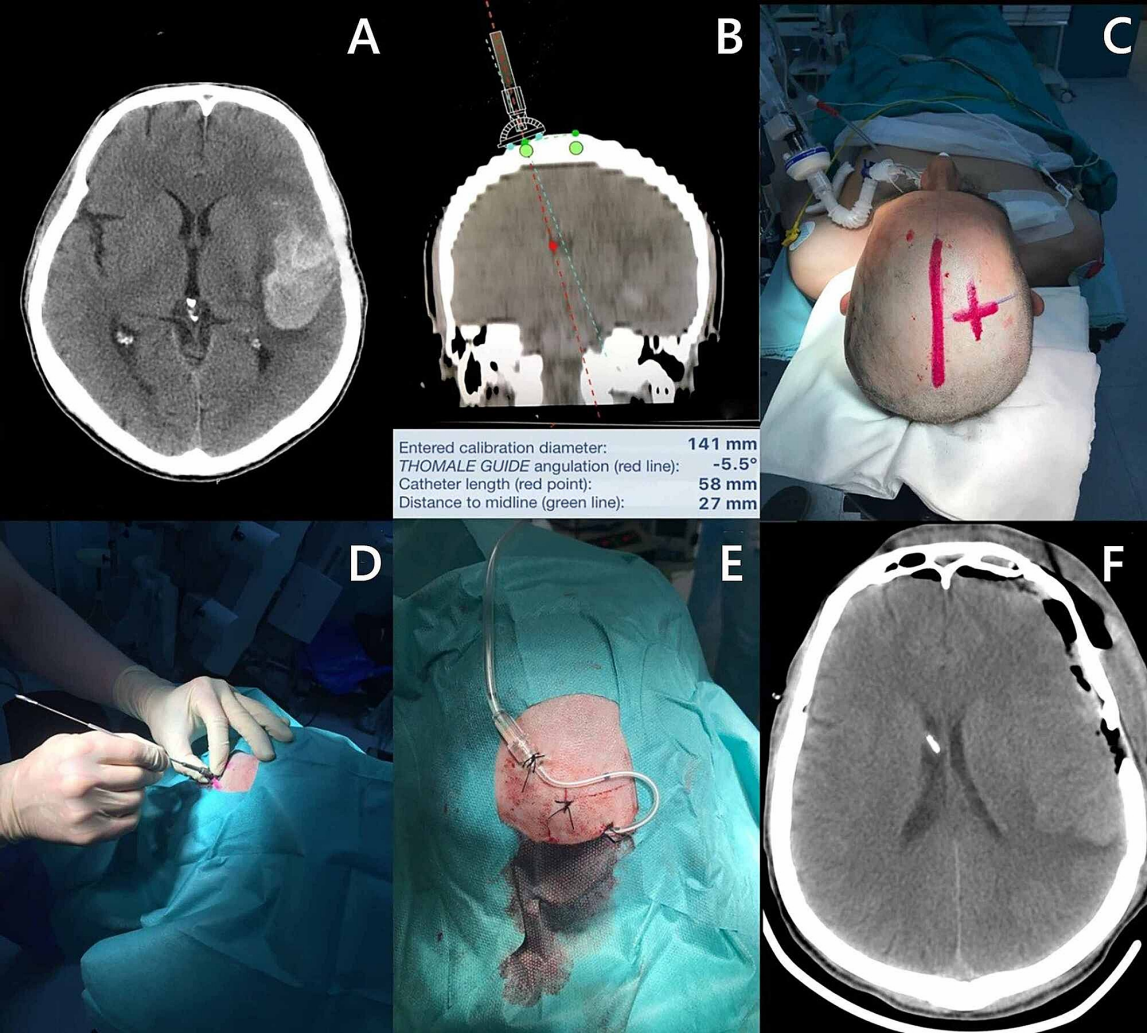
Installation of EVD The images show the various stages in the installation of an EVD into the anterior horn of the right lateral ventricle in a patient on the fourth day after SAH from the left MCA aneurysm using a navigation device A: axial CT of the head at admission. Dislocation of the midline structures of 2 mm and an intracerebral hematoma with a volume of 30 ml were observed; B: calculation of parameters for planning the EVD navigation unit using the Thomale software; C: application of measurements and selection of the trephination point for the installation of EVD; D: installation of EVD as per the Thomale guide; E: the type of postoperative wound; F: CT of the head after surgery EVD: external ventricular drain; SAH: subarachnoid hemorrhage; MCA: middle cerebral artery; CT: computed tomography
